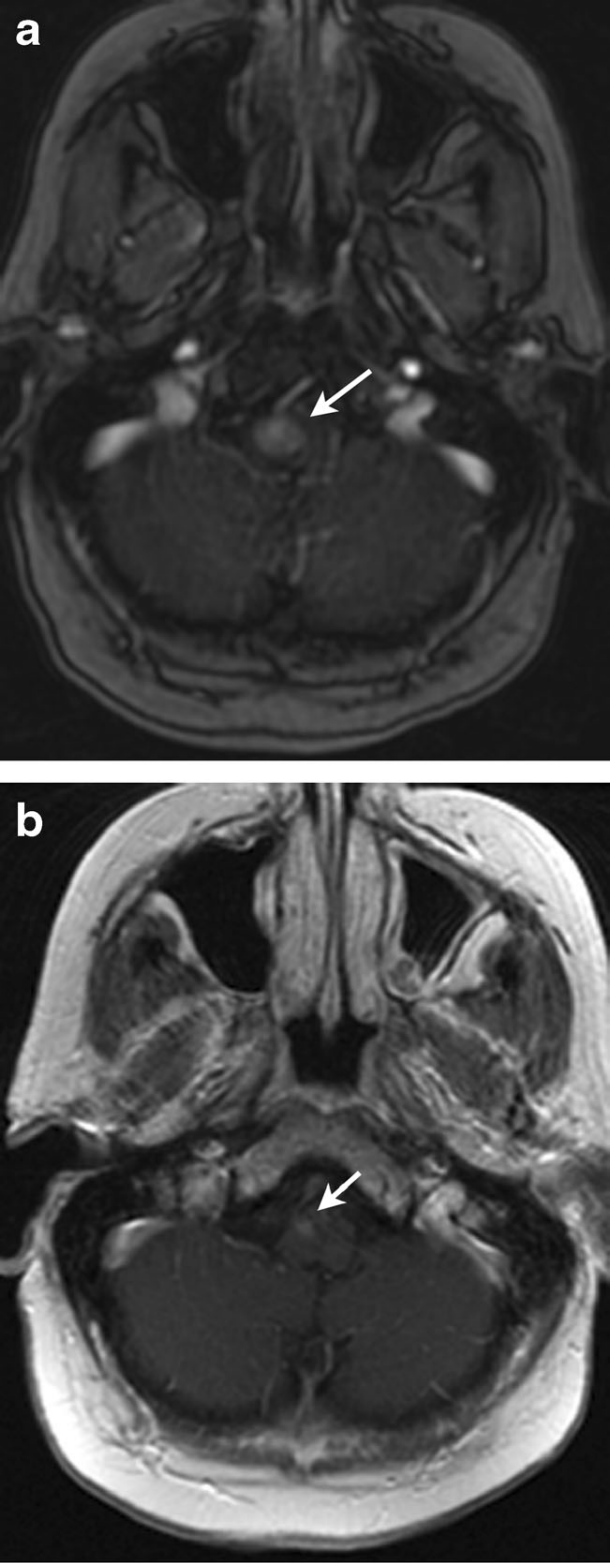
62-year-old woman with metastatic breast cancer and new complaint of numbness and tingling in her left hand and left foot. a Axial contrast-enhanced T1-weighted MR brain image shows homogenously enhancing lesion within the right lateral aspect of the medulla (*arrow*) suggestive of metastasis. b Axial contrast-enhanced T1-weighted MR brain image after whole-brain radiation and 2 months of therapy with lapatinib shows significant interval decrease in size of enhancing lesion in medulla (*arrow*)
